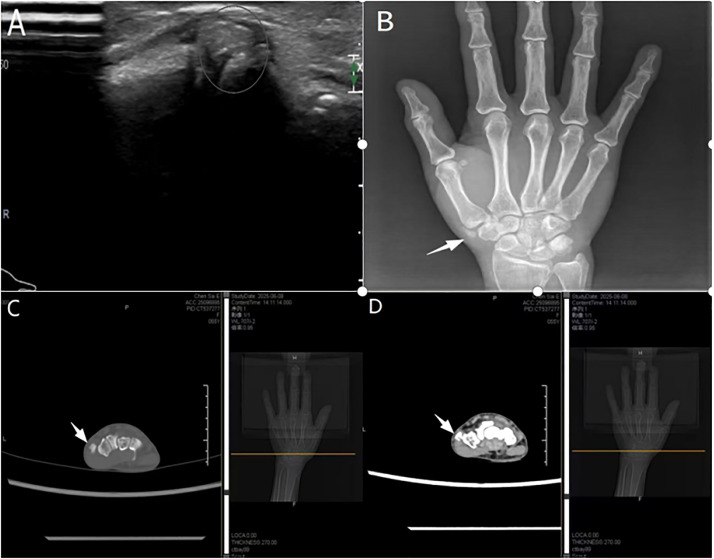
Before treatment radiographic findings. (A) Ultrasound image showing a hyperechoic mass (at the circle). (B) X-ray image showing well-defined calcification deposits in close proximity to the right extensor pollicis tendon (white arrow). (C) CT cross-sectional image of a window of bone displaying calcifications (white arrow). (D) CT cross-sectional image of a window of tissue displaying calcifications (white arrow).
